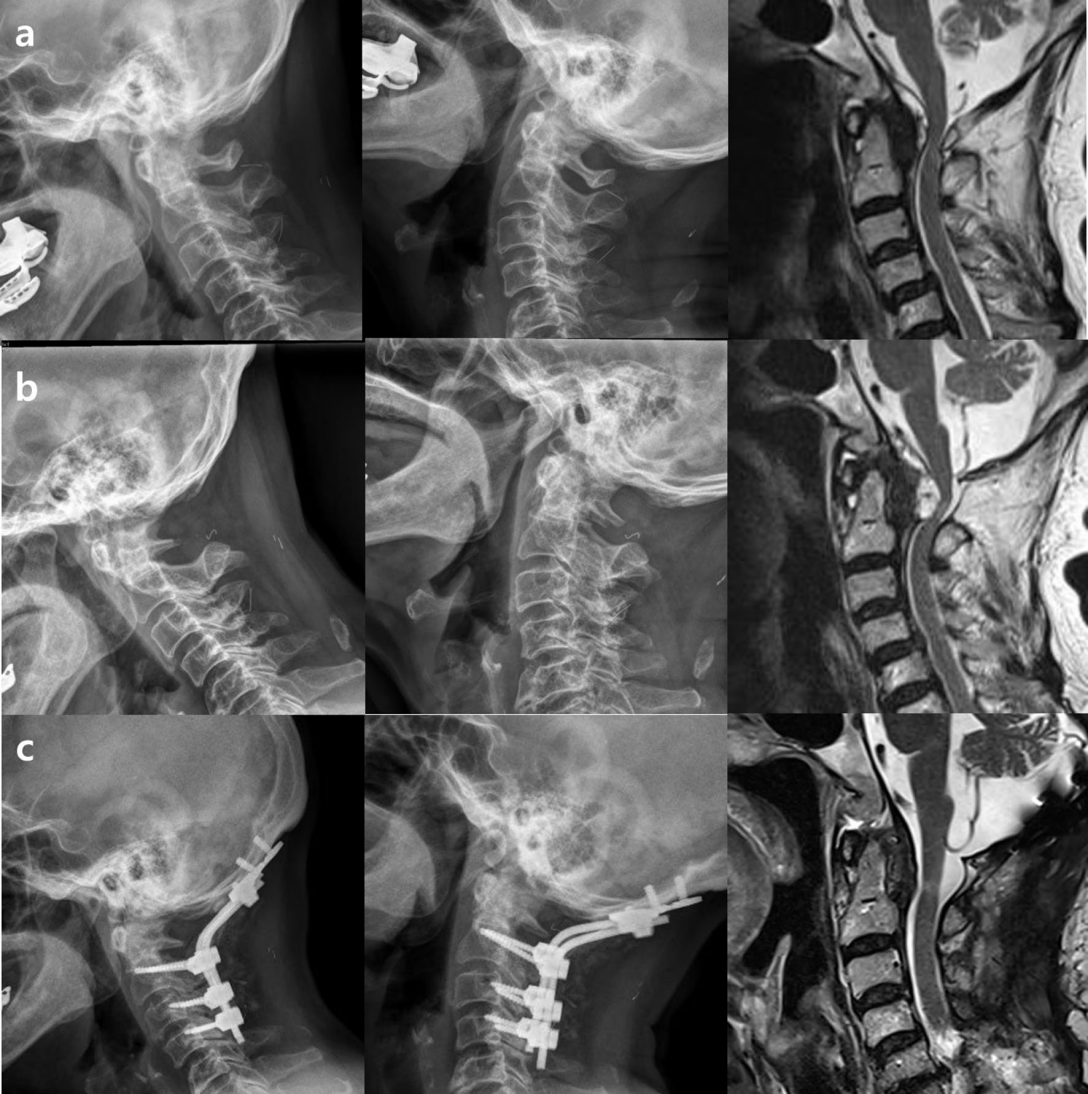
(a) Preoperative MRI revealing a cystic retro-odontoid mass compressing the spinal cord. (b) Immediate postoperative MRI shows a cystic retro-odontoid mass. The degree of spinal cord compression slightly decreased. (c) The cystic mass completely disappeared at the 6-month follow-up visit. *MRI* magnetic resonance imaging.
